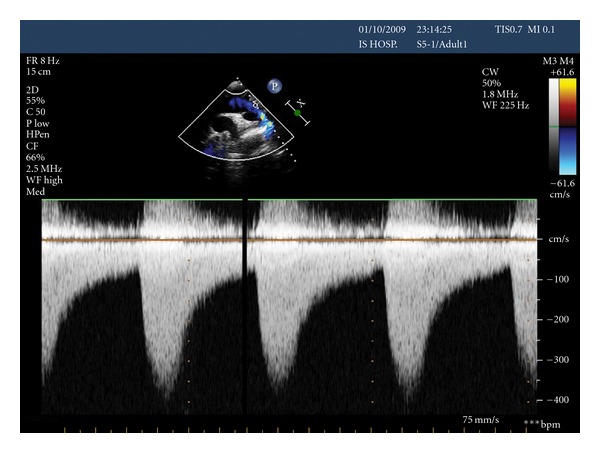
Doppler echocardiography demonstrating the coarctation gradient with a peak flow velocity in the descending aorta of 4.0 m/s, comparable to a gradient of 64 mmHg.
